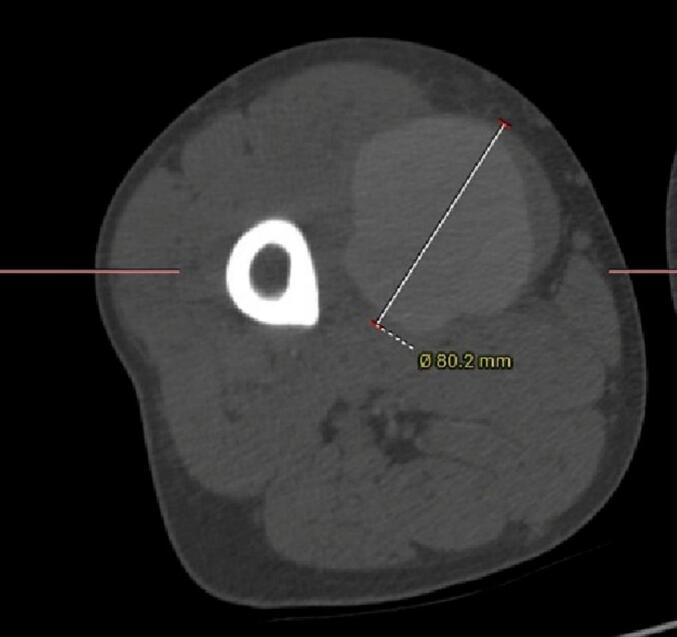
A CT angiography of right the lower limb: Axial view demonstrating a giant pseudoaneurysm of the right SFA with a maximal diameter of 80.2 mm.
